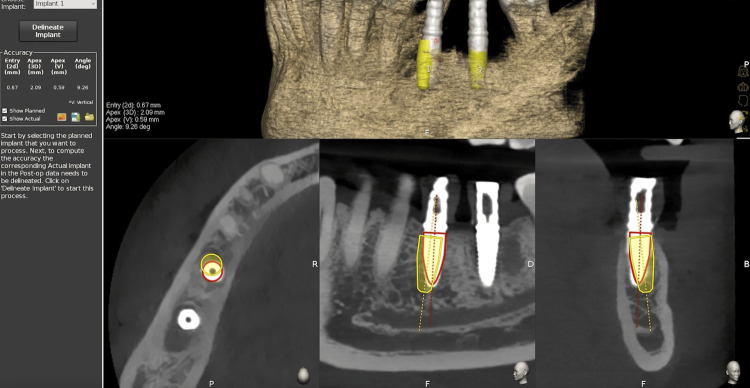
The EvaluNav analysis and CBCT merging CBCT: cone beam computed tomography
